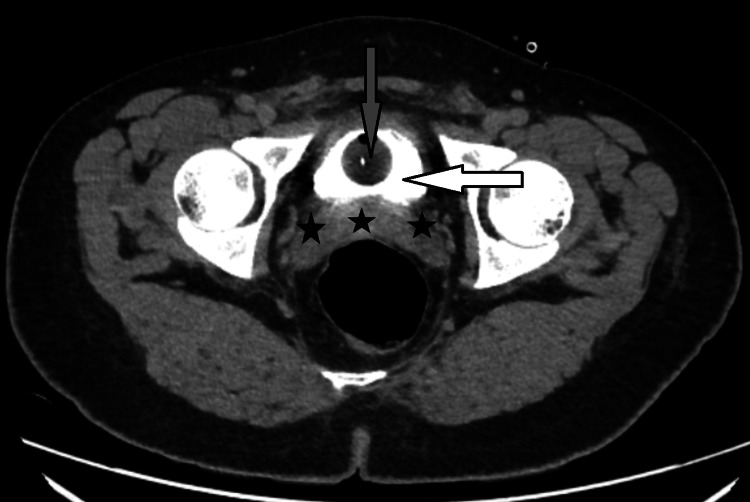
CT abdomen axial view (10-minute delay sequence) day 3 after surgery showing contrast in the bladder (white arrow) with no contrast leak in the peri-vesical area (black stars) with a Foley catheter (gray arrow) in place. White arrow: urinary bladder with contrast, gray arrow: Foley catheter, black stars: peri-vesical area with no contrast leak CT: computerized tomography
